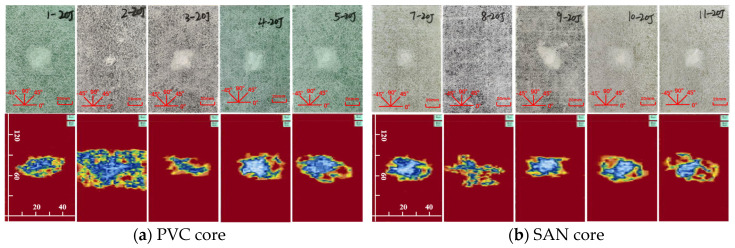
Physical picture of 20 J impact damage and ultrasonic C-scan image.
